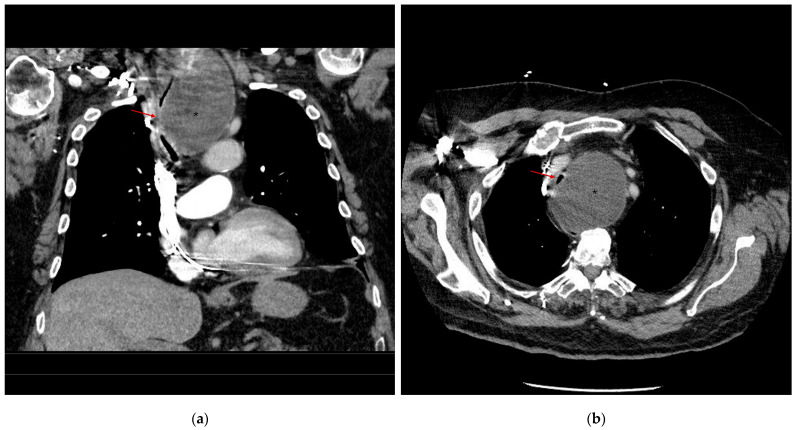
Initial CT imaging of the (a) coronal plane and (b) axial plane: compression of the trachea (arrow: minimum diameter of 3 mm/subtotal collapse) due to the cyst (*), and a shift in anterior mediastinal structures to the right side of the patient.
